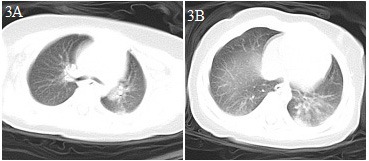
Chest CT images for case 3 showing a patchy dense shadow in the posterior part of the left lung with a blurred boundary on day 2 after symptom onset (A,B).
